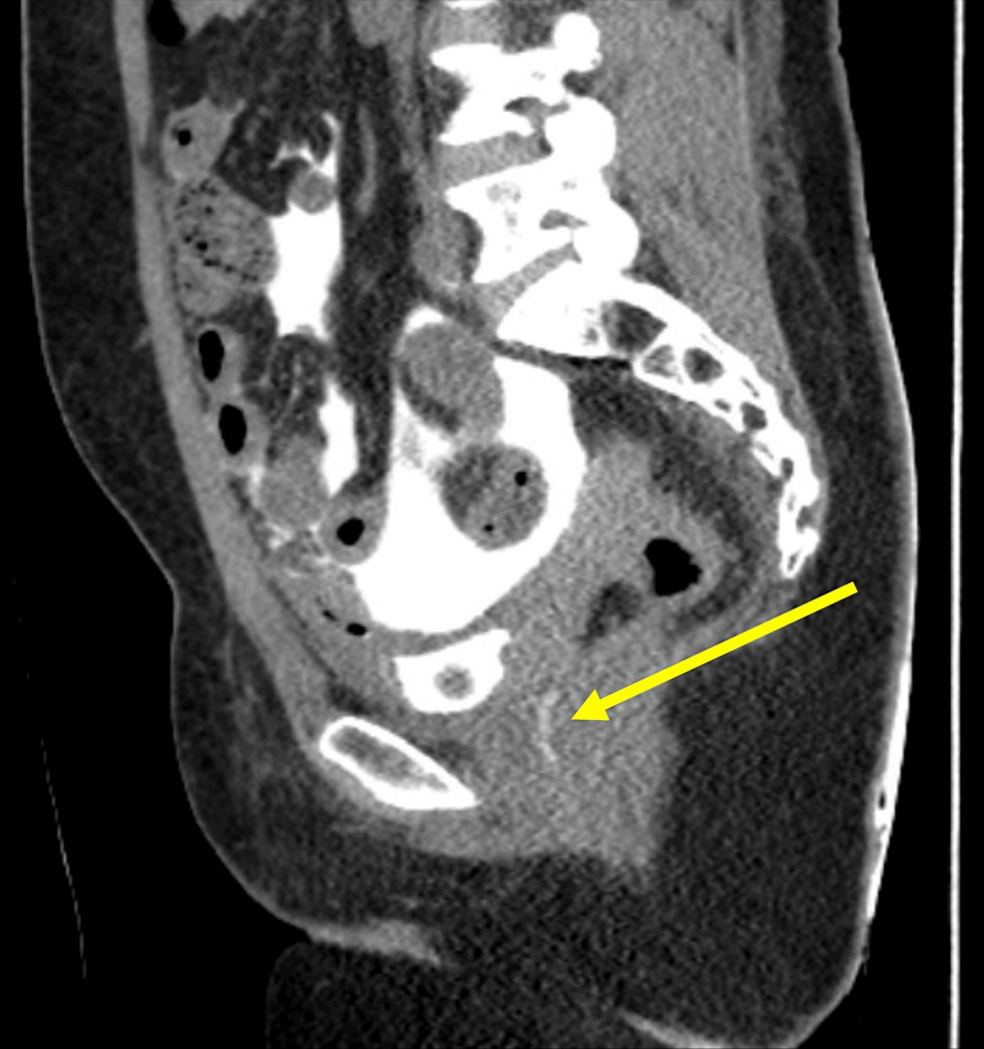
CT cystogram demonstrating leakage of contrast (yellow arrow) from the bladder into the vagina. CT: computed tomography
